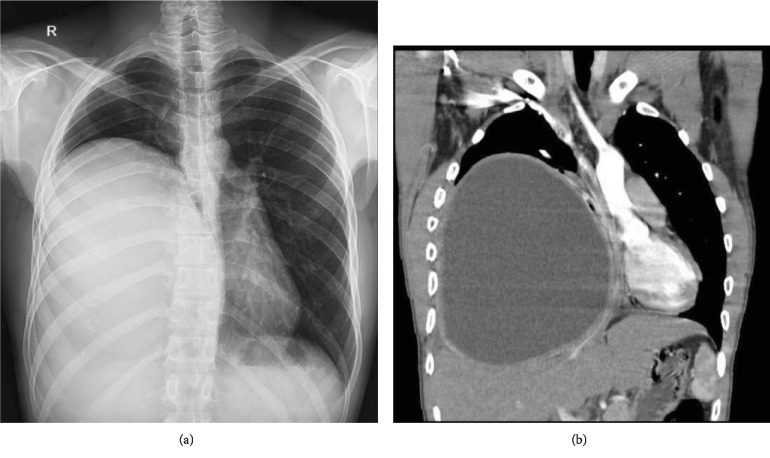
(a) Chest X-ray and (b) computed tomography (coronal view) on admission revealing a large pulmonary hydatid cyst in the right lower lobe.
